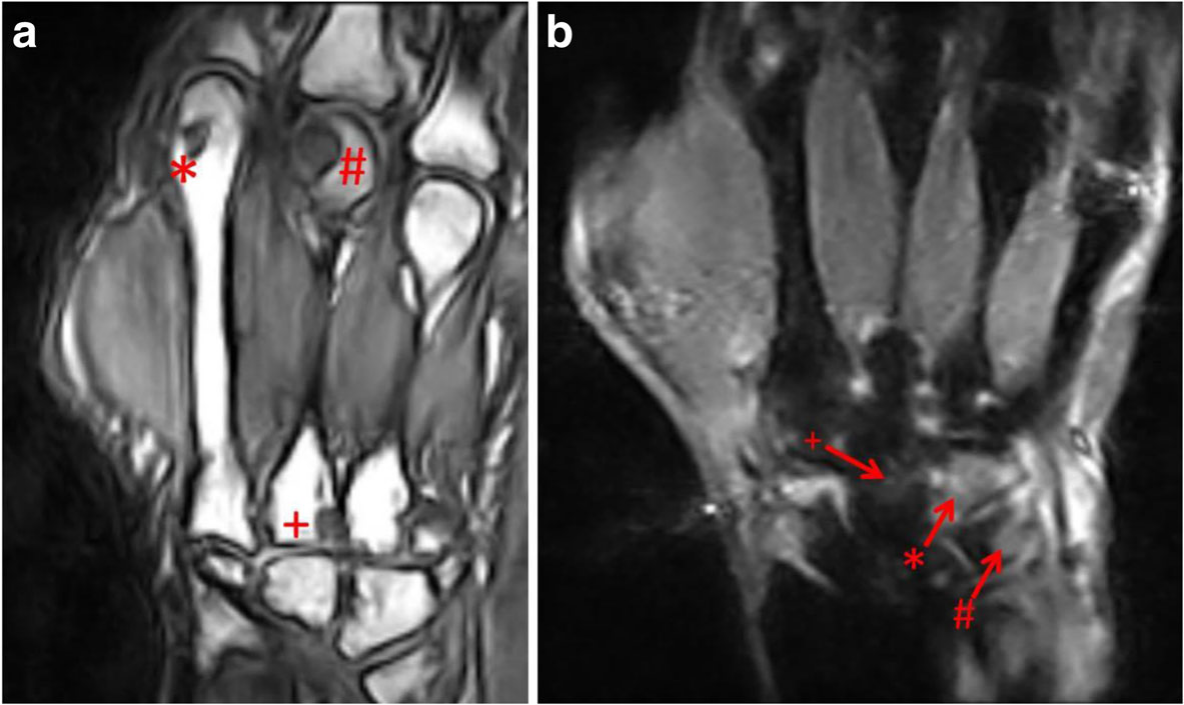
a Nativ T1-weighted image in coronal orientation. Erosion grade 1 in the head of metacarpus D2 (_*_), erosion grade 3 in the head of metacarpus 3 (#). Additionally, erosion of the basis of metacarpus 3 (+). The RAMRIS score of this patient was 48. b Short-Tau-Inversion-Recovery Sequenz (STIR) in coronal orientation: Osteoedema in Os hamatum (*arrow with*
